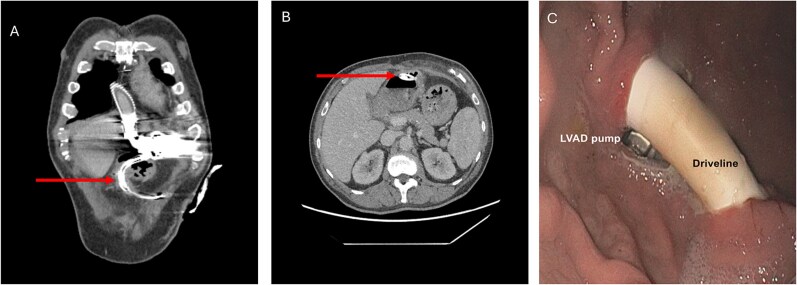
Preoperative CT scan (A, B) and esophagogastroduodenoscopy (C) revealing intragastric DL transposition. The arrow points at the DL.
